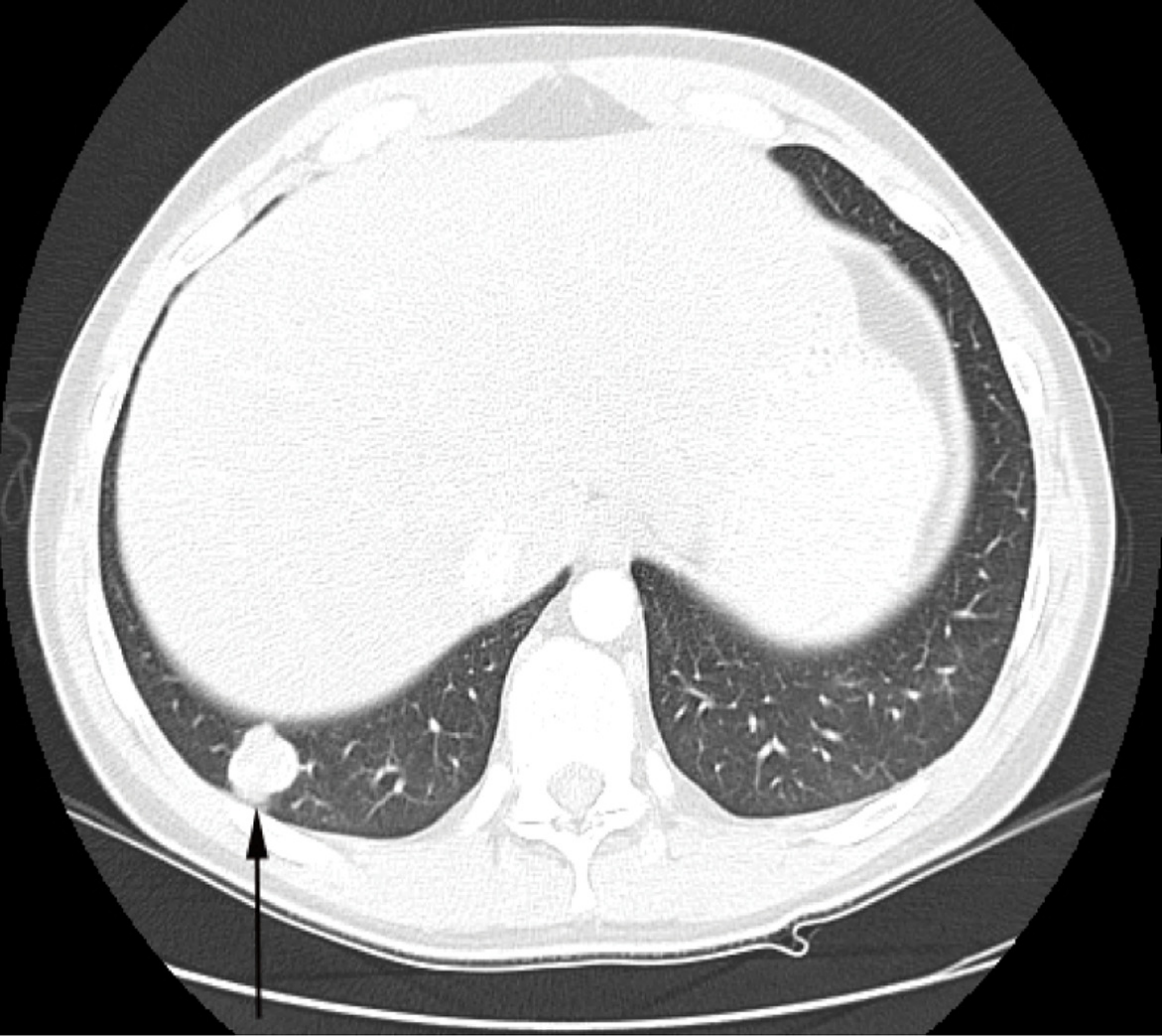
An axial CT image of the chest, with intravenous contrast, demonstrates a 2.3-cm right basilar nodule (arrow), confirmed to be a metastatic focus. Multiple additional smaller pulmonary nodules were seen throughout the lung parenchyma (not shown).
